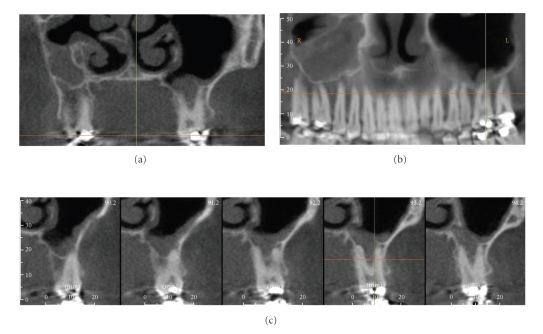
On conventional intraoral periapical radiography periapical mucositis (PM) presents as a relatively radiopaque, soft-tissue, dome-shaped lesion localized to the apex of a maxillary posterior tooth and projecting into the floor of the maxillary sinus. Most are indistinguishable from mucosal lesions of intrinsic sinus origin such as antral mucosal pseudocysts (see Figure 9). While clinically asymptomatic, they are usually associated with necrotic or failing root canal-filled teeth. PM is a localized mucosal thickening of the sinus membrane, secondary to a breach of periradicular inflammation, and will resolve after successful endodontic treatment. This patient gave a history of persistent left side pain over the maxillary molar region of 4-month duration. Treatment for sinusitis did not relieve the symptoms. Panoramic and intraoral dental radiology did not reveal a cause. Coronal CBCT imaging (a) demonstrates acute sinusitis bilaterally with 50% to 70% opacification and previous uncinectomy and antrostomy (as evidenced by the loss of the superior medial wall of the right sinus) of the right sinus, whereas the left sinus shows thickened mucosal lining with a dome-shaped soft tissue lesion overlying the roots of the restored left maxillary first molar tooth. 5 mm reformatted panoramic (b) and 1 mm cross-sectional (c) reconstructions of the maxillary left first molar show periapical lesional penetration and communication with the floor of the sinus in this region. Data acquired on an iCAT, Imaging Sciences International, Hatfield, PA USA at 0.3 mm resolution and reformatted using InVivo Dental, Anatomage, San Jose, CA.
